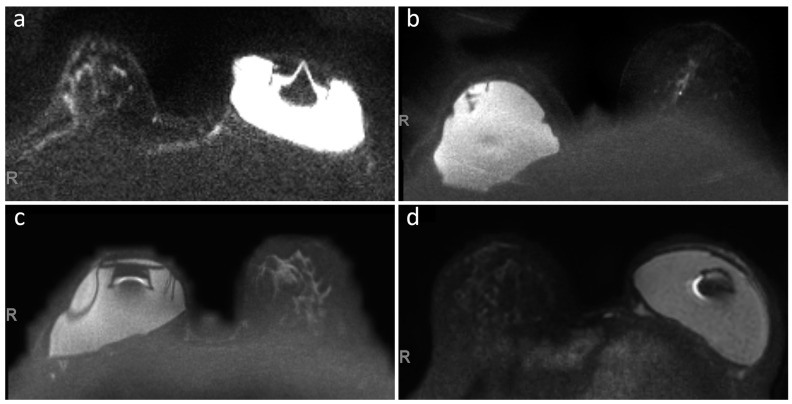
DWI images (*b* value 1000 s/mm²) of four patients showing different levels of overall image quality degradation caused by the presence of unilateral breast tissue expander, as rated by both readers. (a) “Poor” image quality”; (b) “Sufficient” image quality; (c) “Good” image quality; (d) “Excellent” image quality. Of note, in panels (a,b), the high field inhomogeneity makes the expander signal appear particularly hyperintense.
